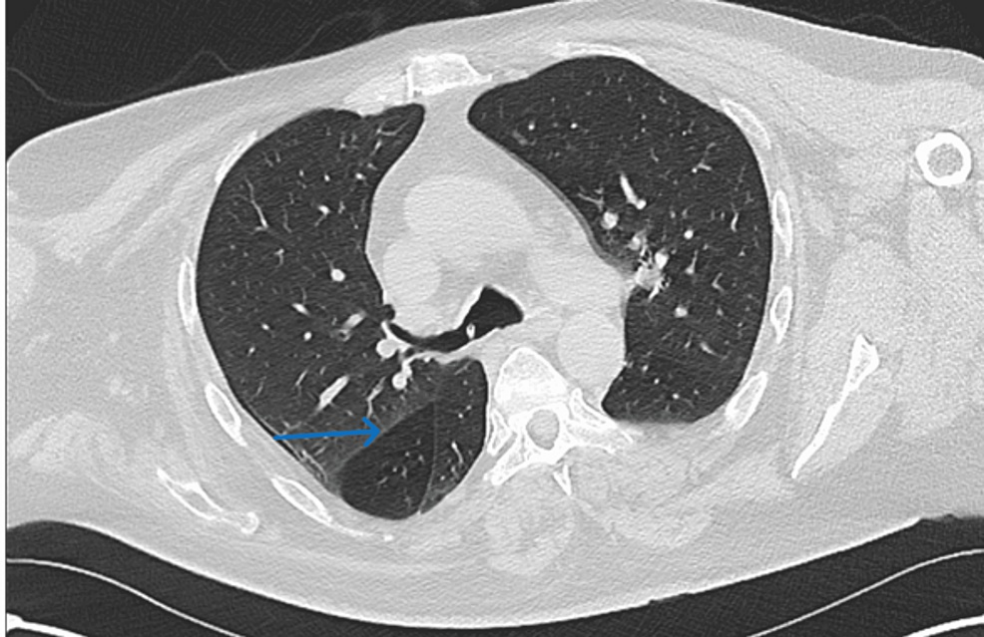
CT scan showing stable appearance of the trachea and right middle and lower lobe bronchi. The partial collapse of the right lobe was attributed to mucus plugging
